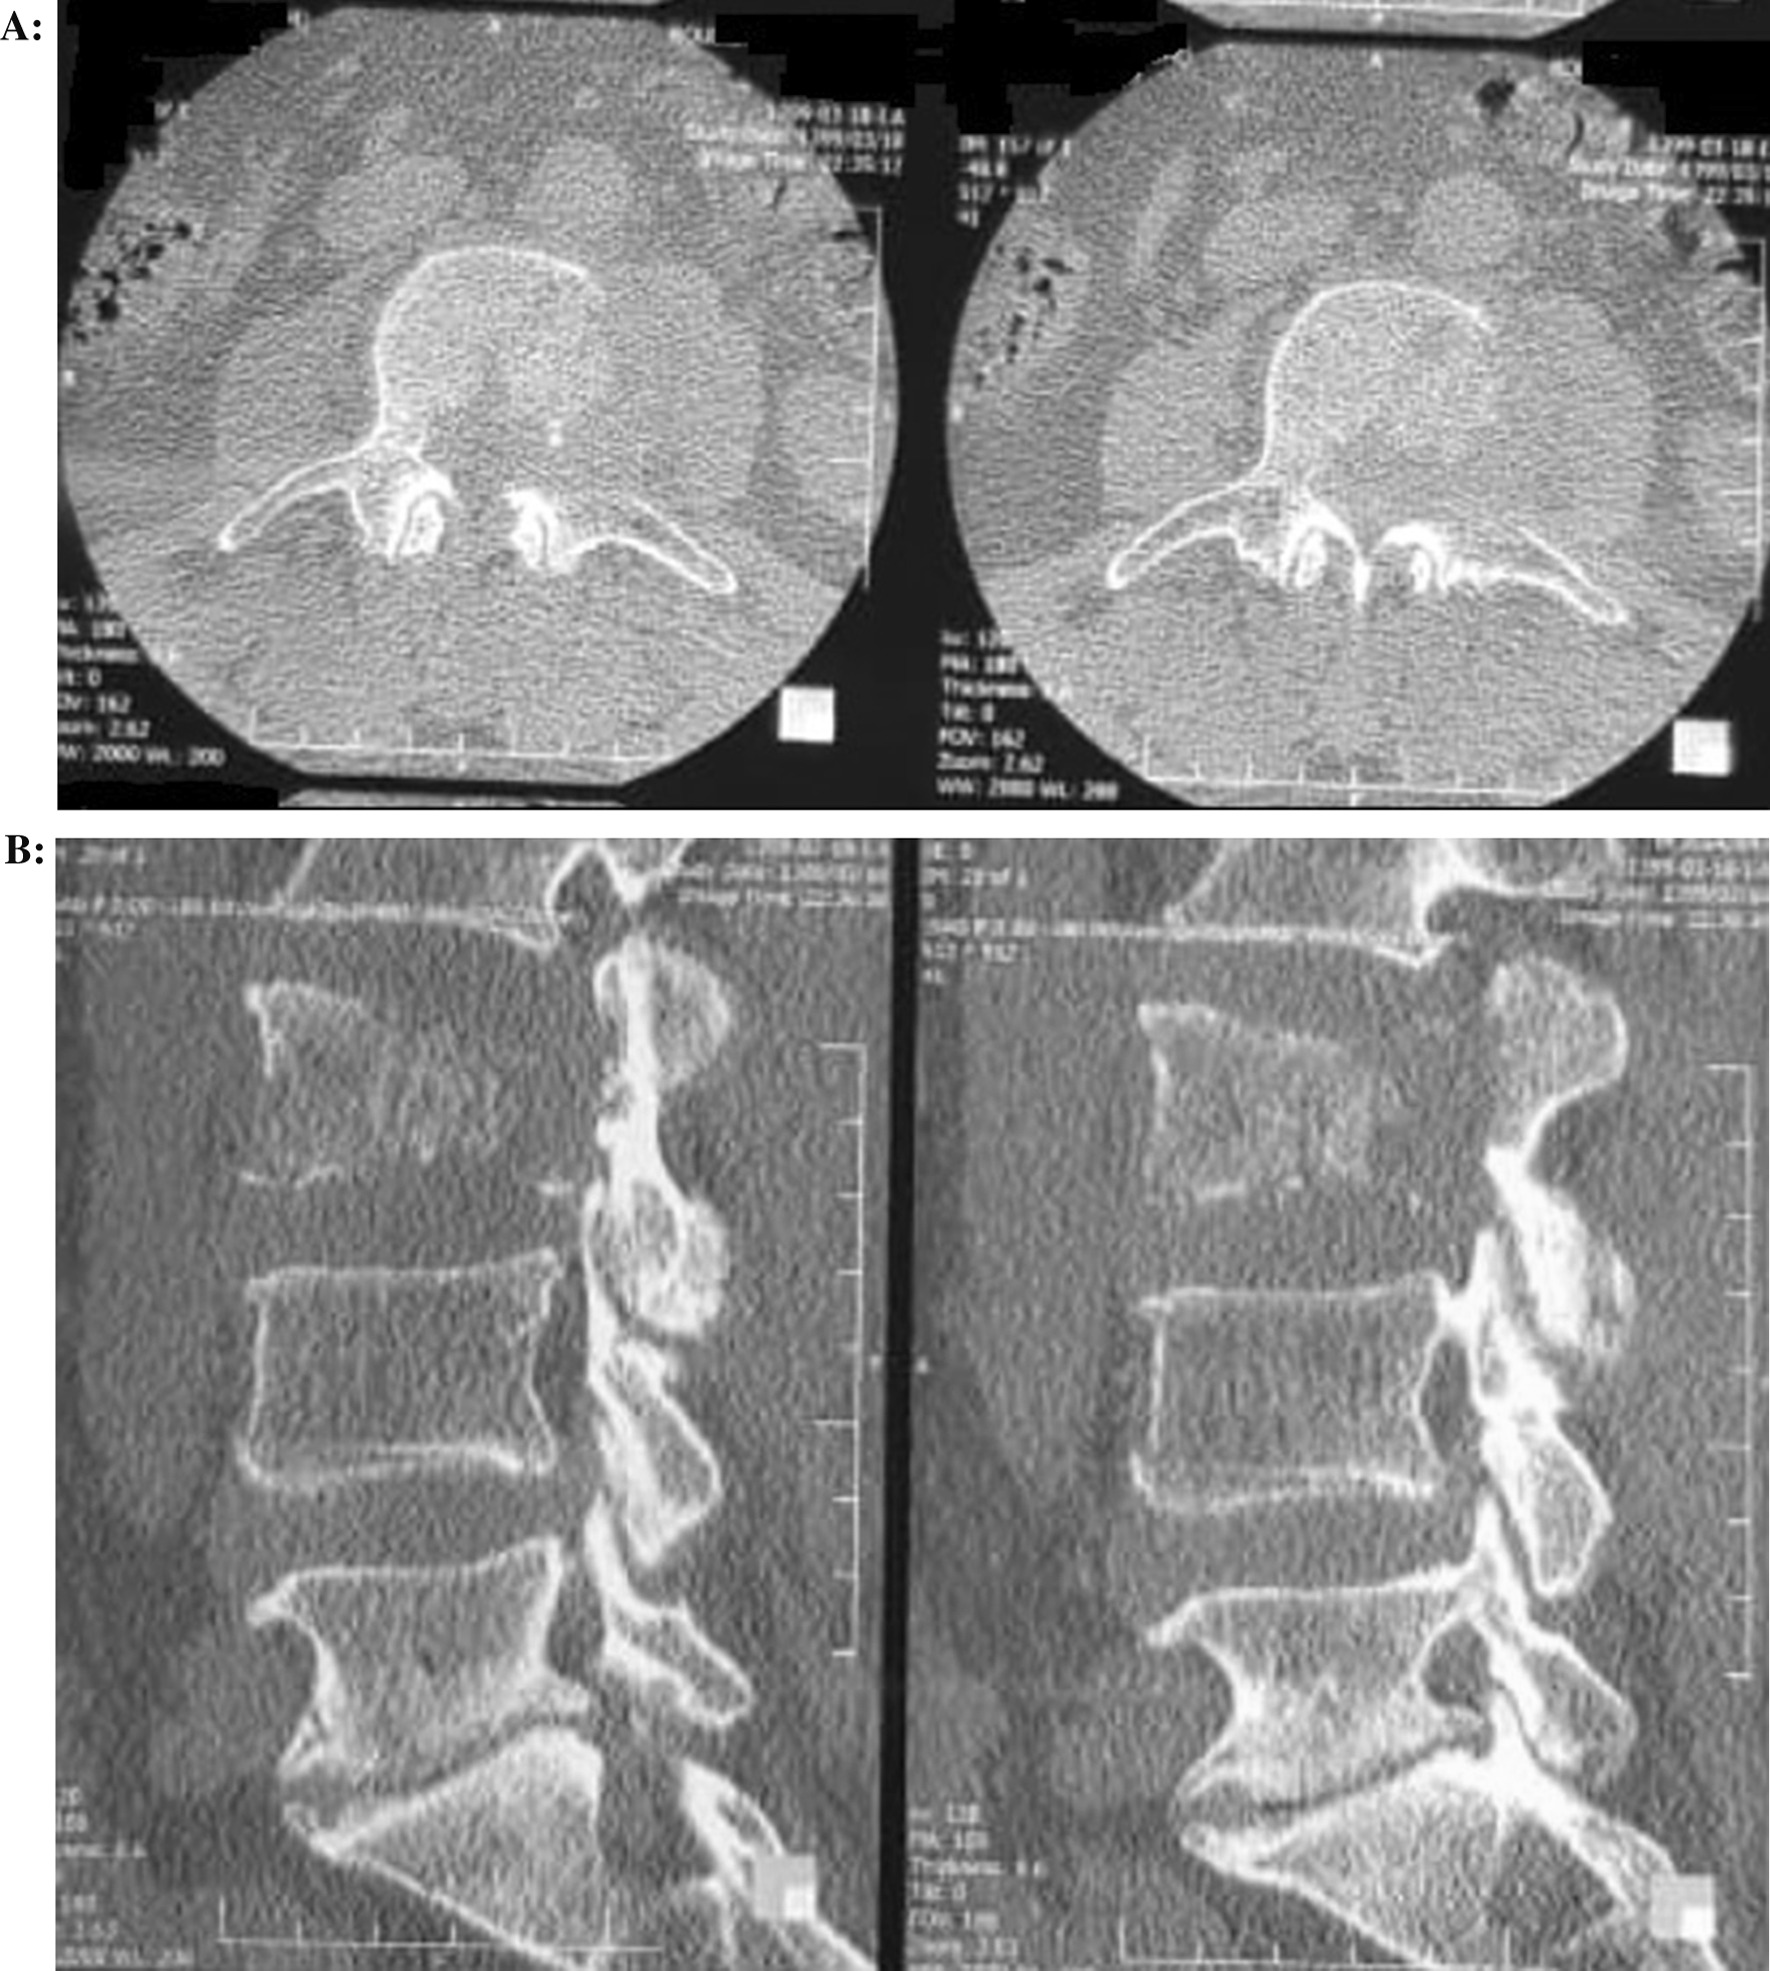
Axial (A) and sagittal (B) computed tomography images showing bony destruction on L3 vertebra
